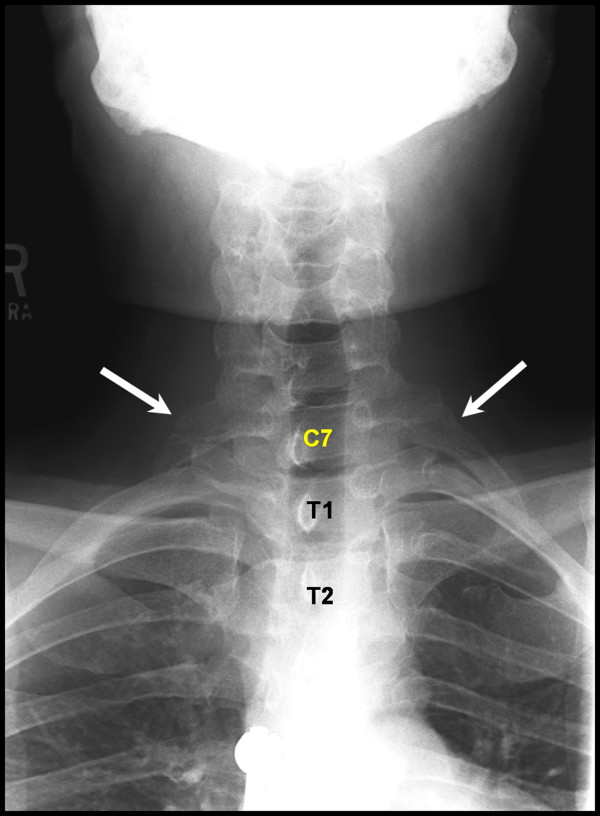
Cervical ribs at C7. Plain antero-posterior radiographs demonstrate C7 vertebra bearing a pair of ribs, left larger than right, which cannot be considered thoracic because they do not articulate with the manubrium.
